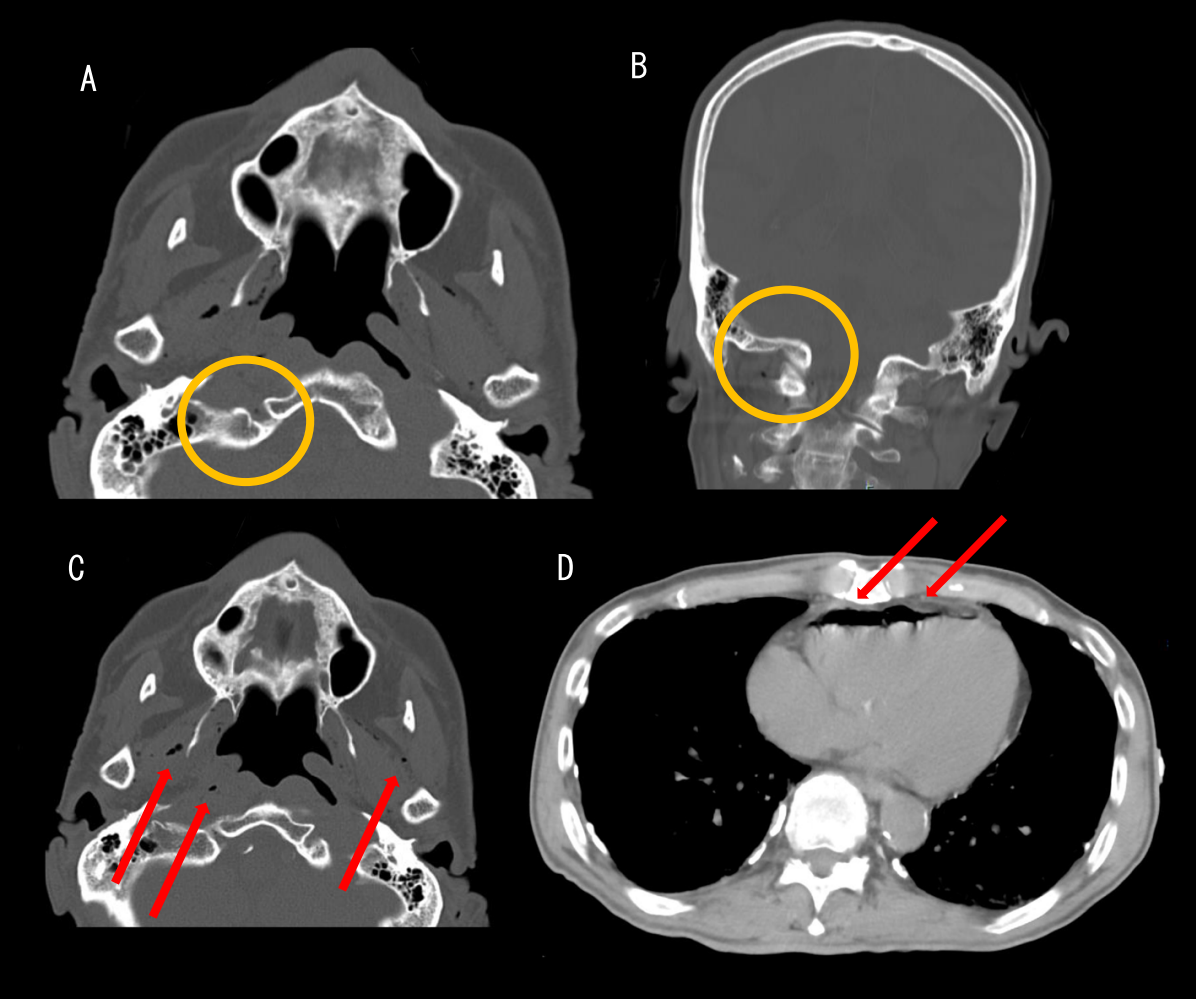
CT scans obtained during trauma evaluation. a Head axial CT showed basilar skull fracture of the occipital bone (circle). b Head coronal CT showed basilar skull fracture of the occipital bone (circle) as well as a. c Head axial CT showed air in the vein at the head and neck (arrow). d Chest CT showed air in the right ventricle (arrow)
